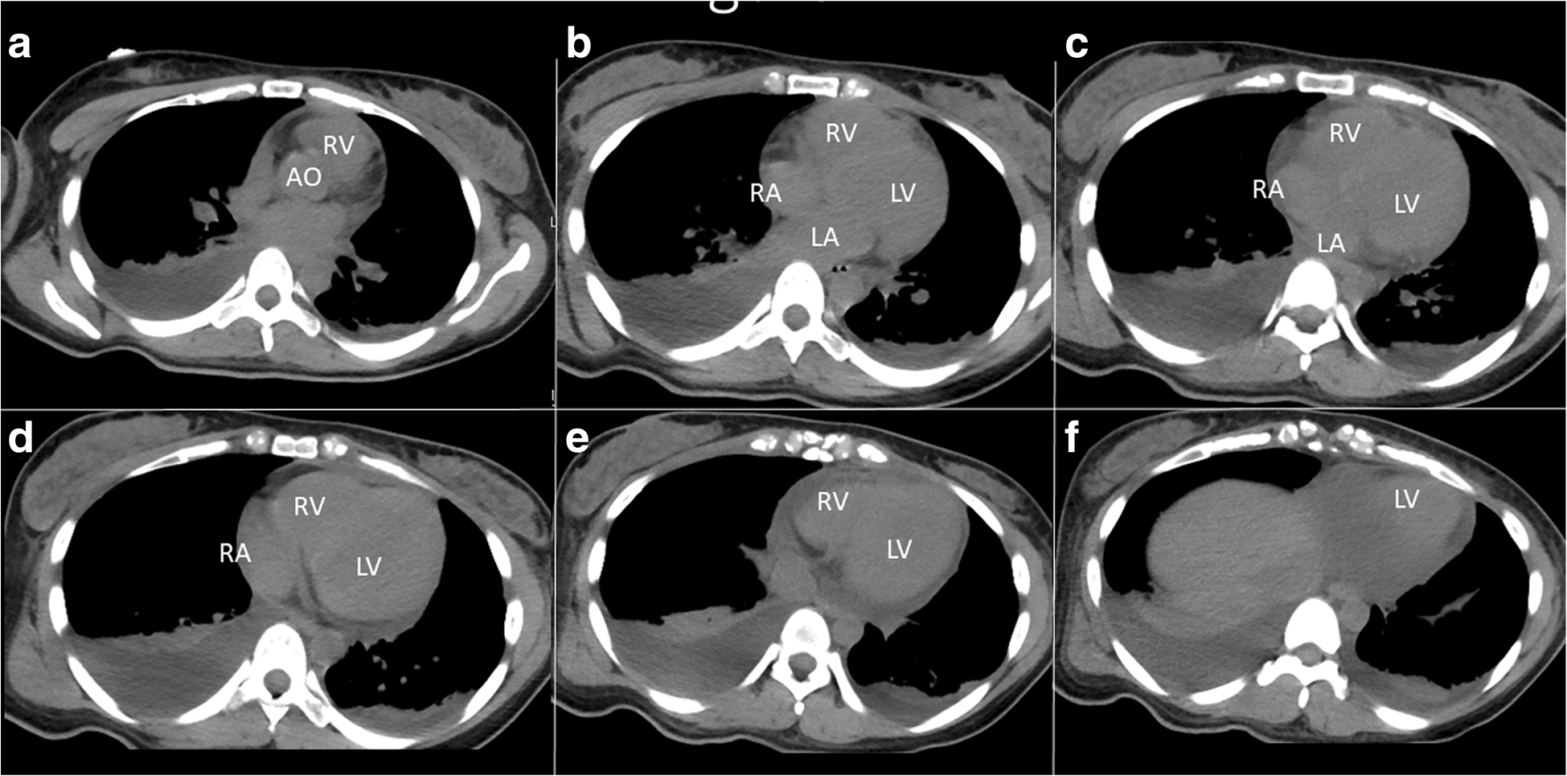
Computed tomography of the heart. (a-f) Consecutive slices in horizontal view. Scans revealed a minimal pericardial effusion. Ao, ascending aorta; RA, right atrium; RV, right ventricle; LA, left atrium; LV, left ventricle
